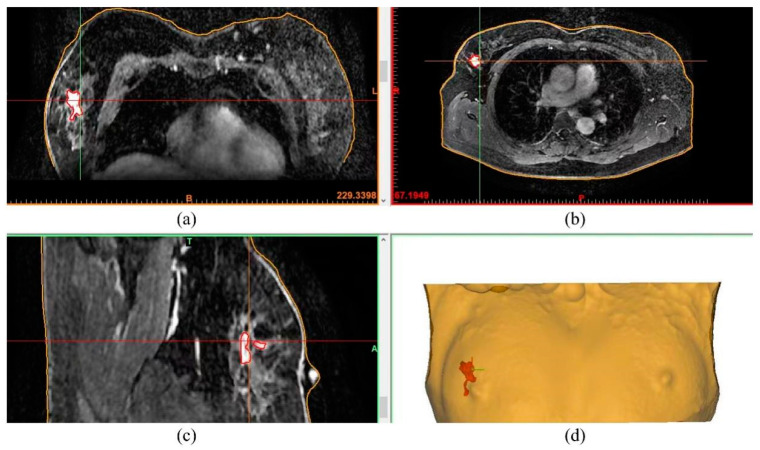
Supine MRI images showing the tumour’s location and margin marked as the red contours: (a) axial view; (b) coronal view; (c) sagittal view. (d) 3D model reconstruction of the tumour and surrounding tissues based on the MRI image series. The red patch depicts the tumour.
